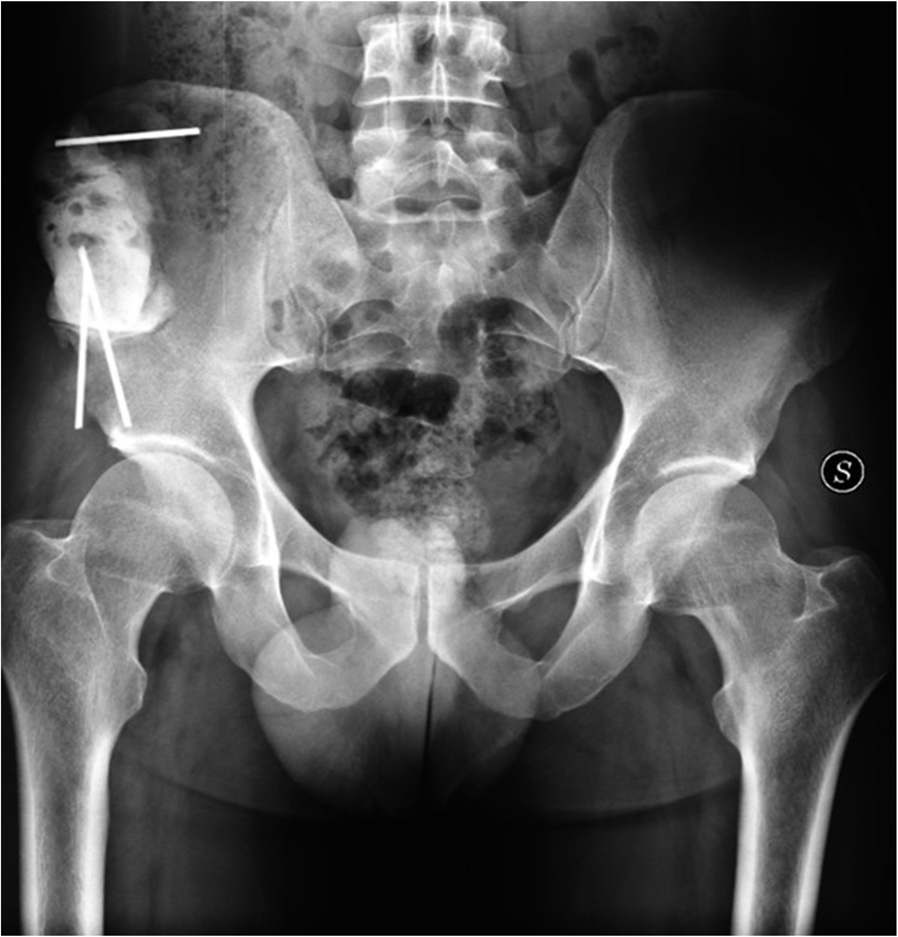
One-year post-operative X-ray.
